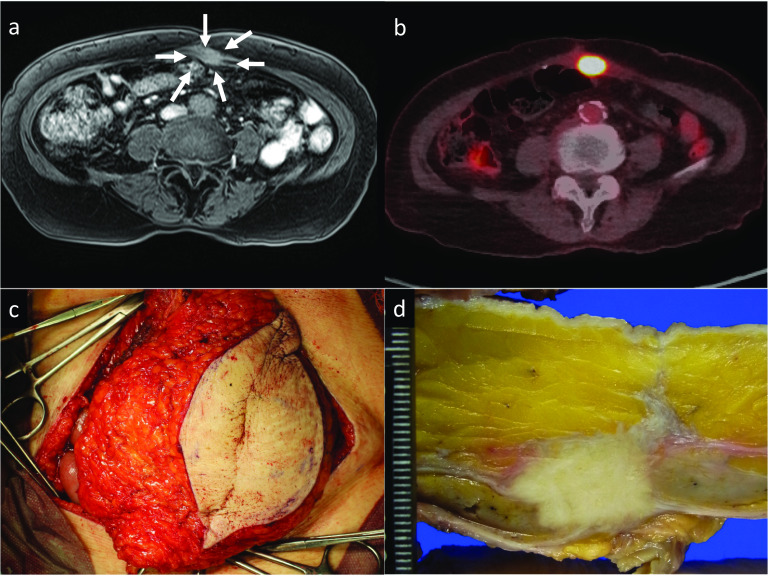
Imaging and intraoperative and gross findings of the second abdominal wall recurrence. a Contrast-enhanced abdominal magnetic resonance imaging showed a 15-mm iso-intensity signal localized in the abdominal wall in T1-weighted imaging that was suggestive of recurrence (white arrows). b
